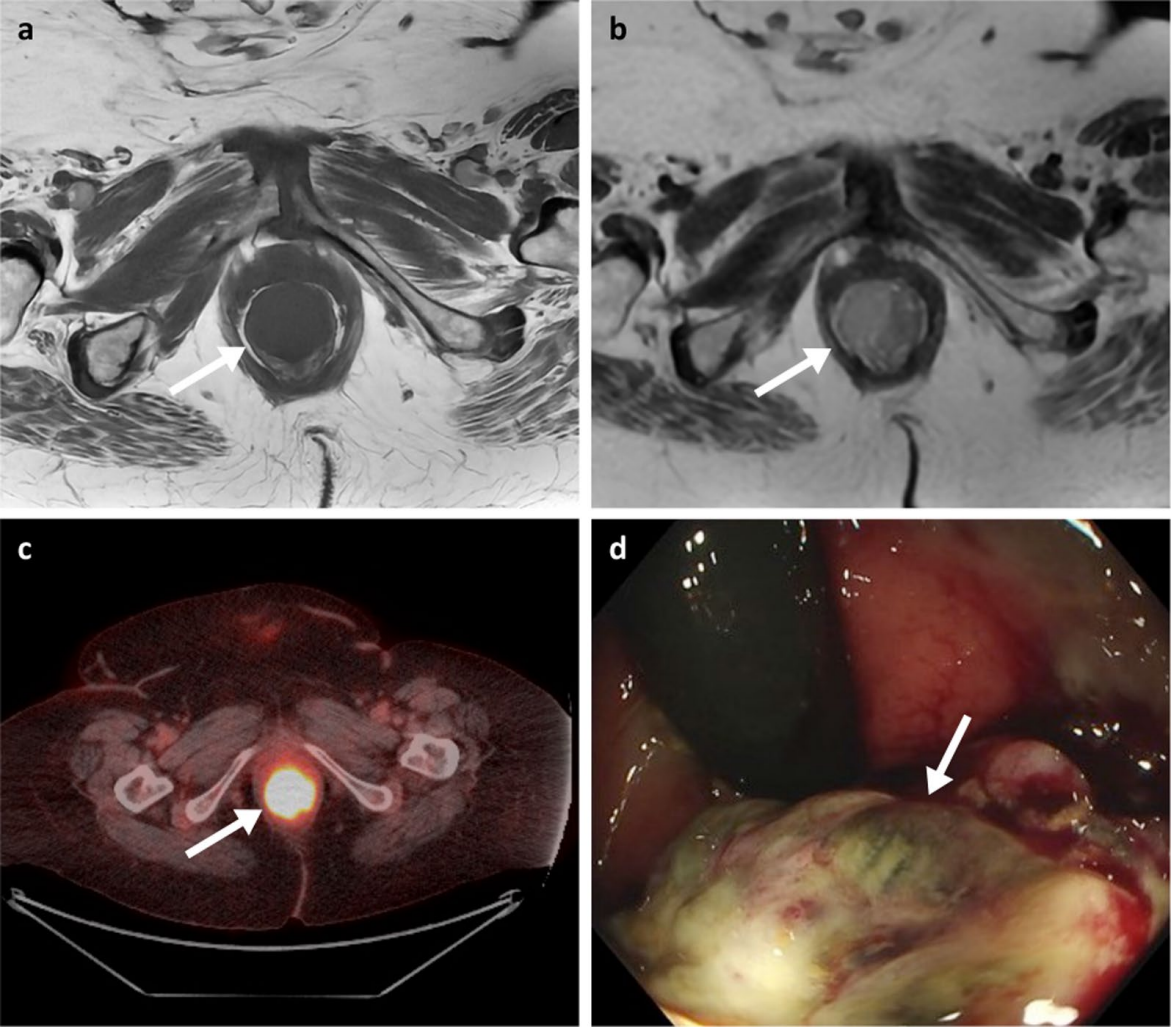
MRI T1WI and T2WI axial (a, b), PET-CT axial (c) and flexible sigmoidoscopy (d) of a 71-year-old female patient with a metastatic melanoma anorectal mass (arrows). Black pigment is visible within the ulcerated part of the lesion on sigmoidoscopy
